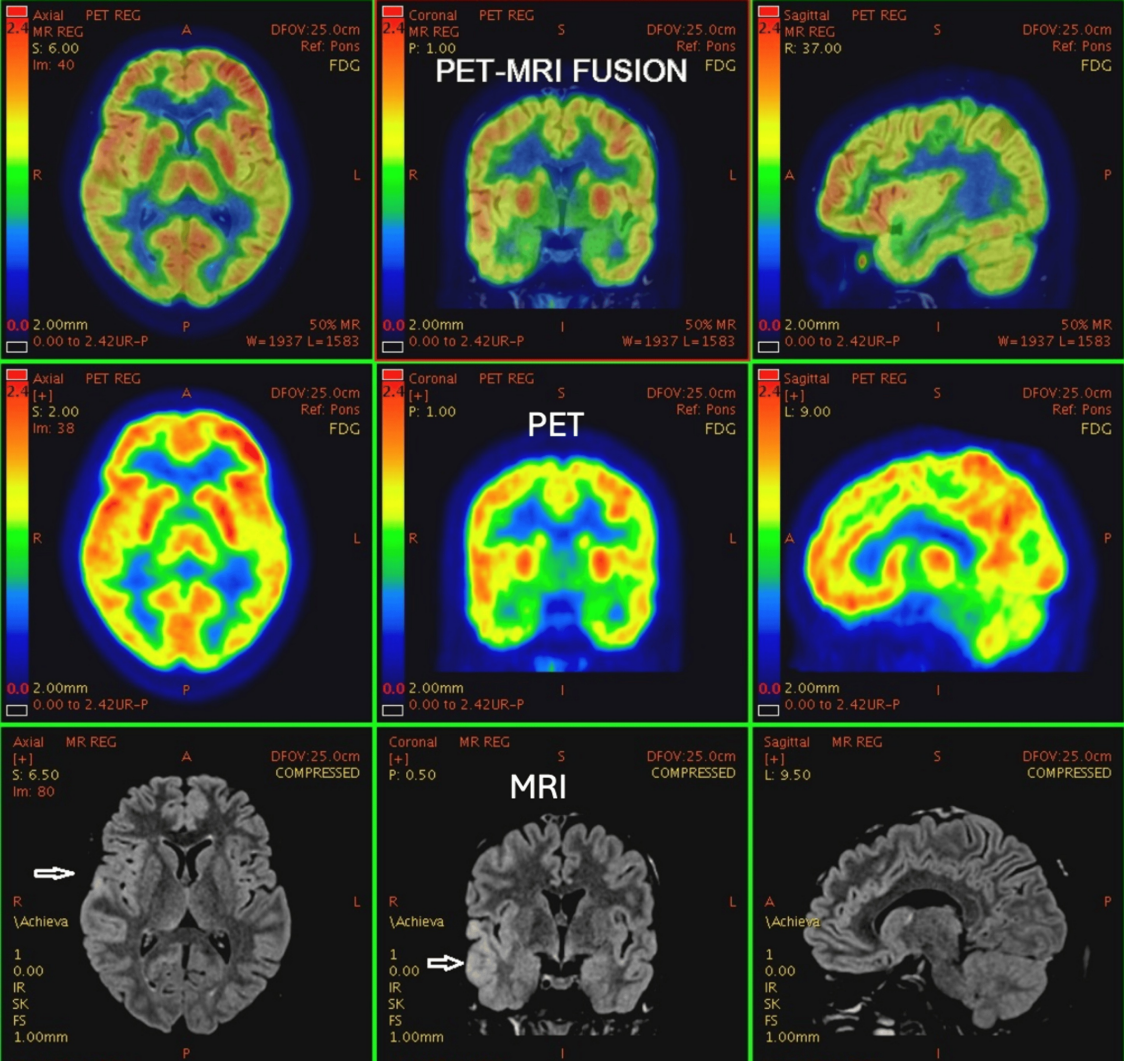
27-year-old female, K/c/o seizure disorder on treatment. C/o staring looks, irrelevant talk, bradycardia, and headache for 7-10 days. PET-MRI study shows the increased signal on FLAIR image in the right lateral temporal lobe and right insular cortex with a mild increase in FDG activity - likely to be postictal edema, although similar appearance could be seen in misalignment. PET: positron emission tomography; MRI: magnetic resonance imaging; FDG: fluorodeoxyglucose; FLAIR: 3D fluid-attenuated inversion recovery
